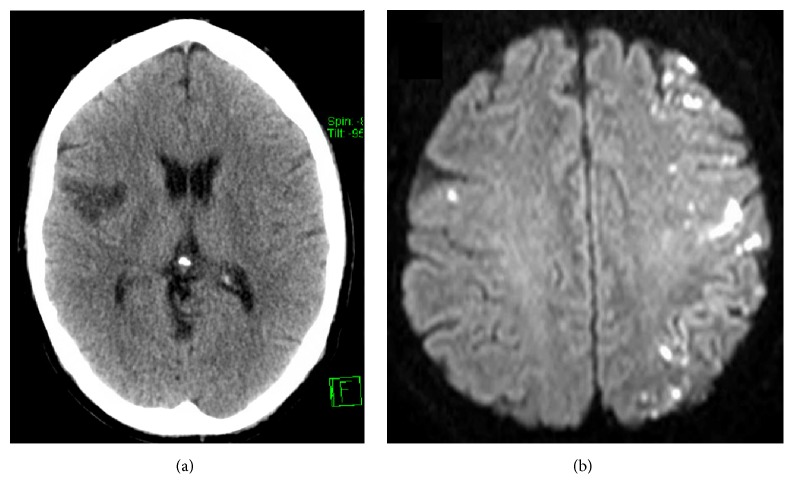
Cerebral infarction in injecting drug users. (a) CT scan showing infarct resulting from paradoxical embolus of injected Zopiclone. (b) MR DWI image of multiple emboli caused by inadvertent injection of diamorphine (Heroin) into left common carotid artery.
